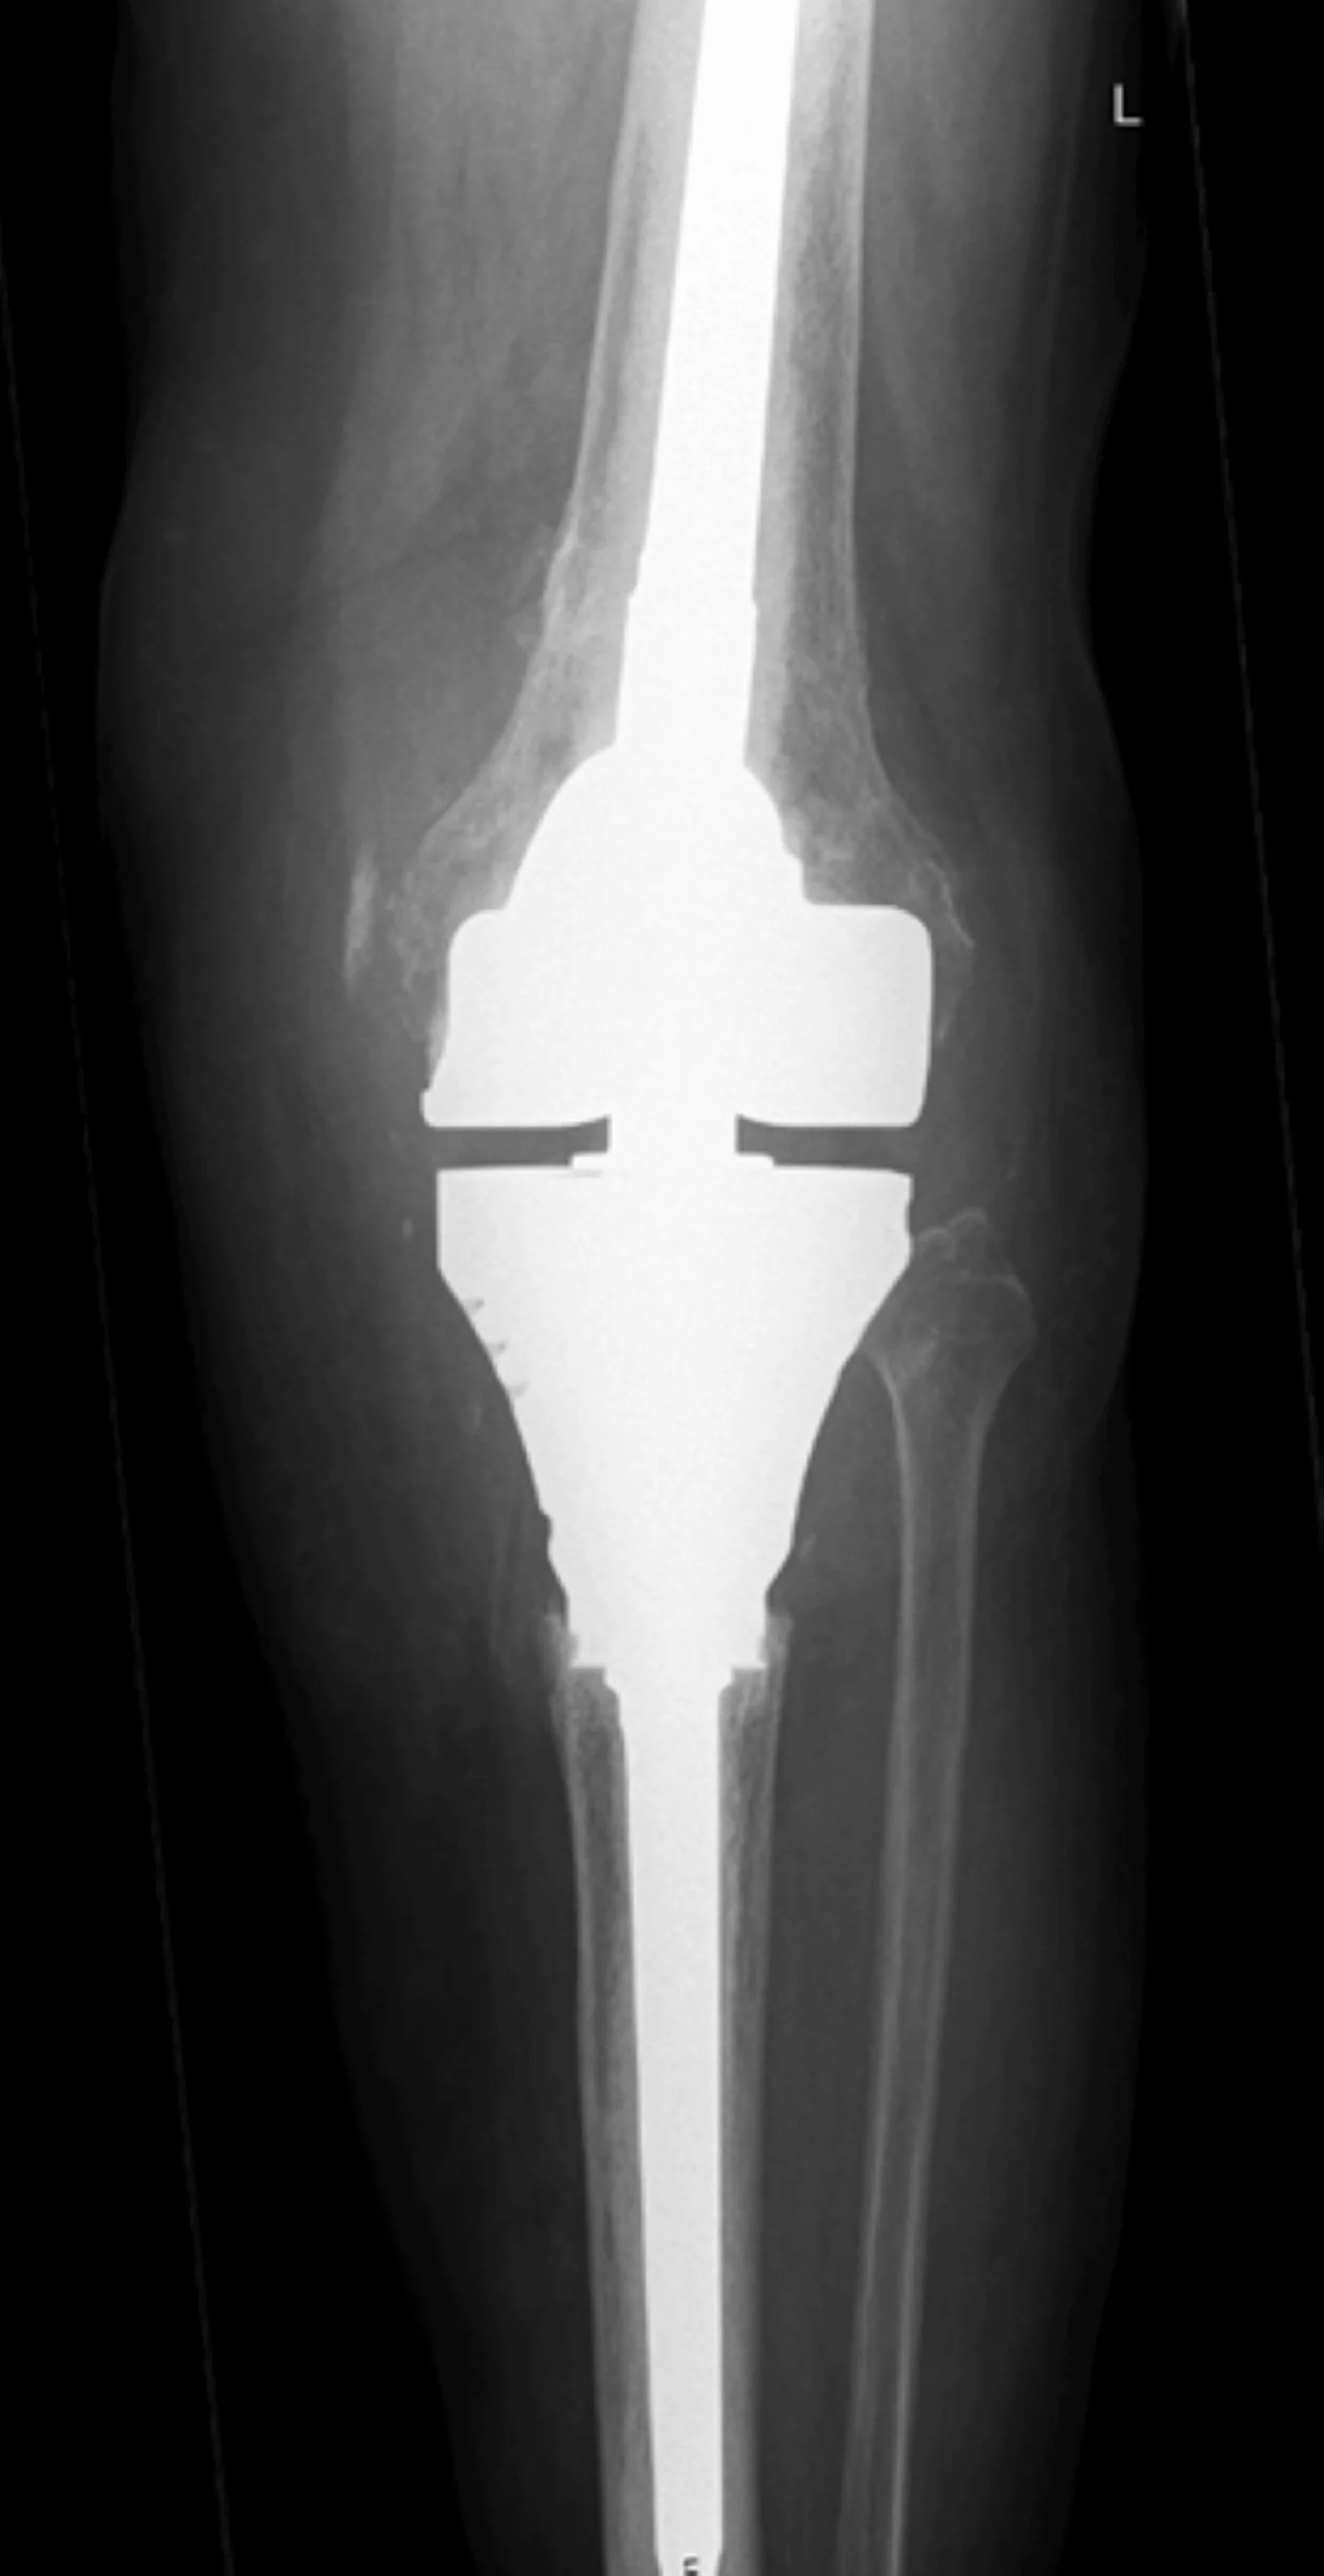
Anteroposterior radiograph of the left knee one year postoperatively showing appropriate implant positioning and no complications after revision arthroplasty.
